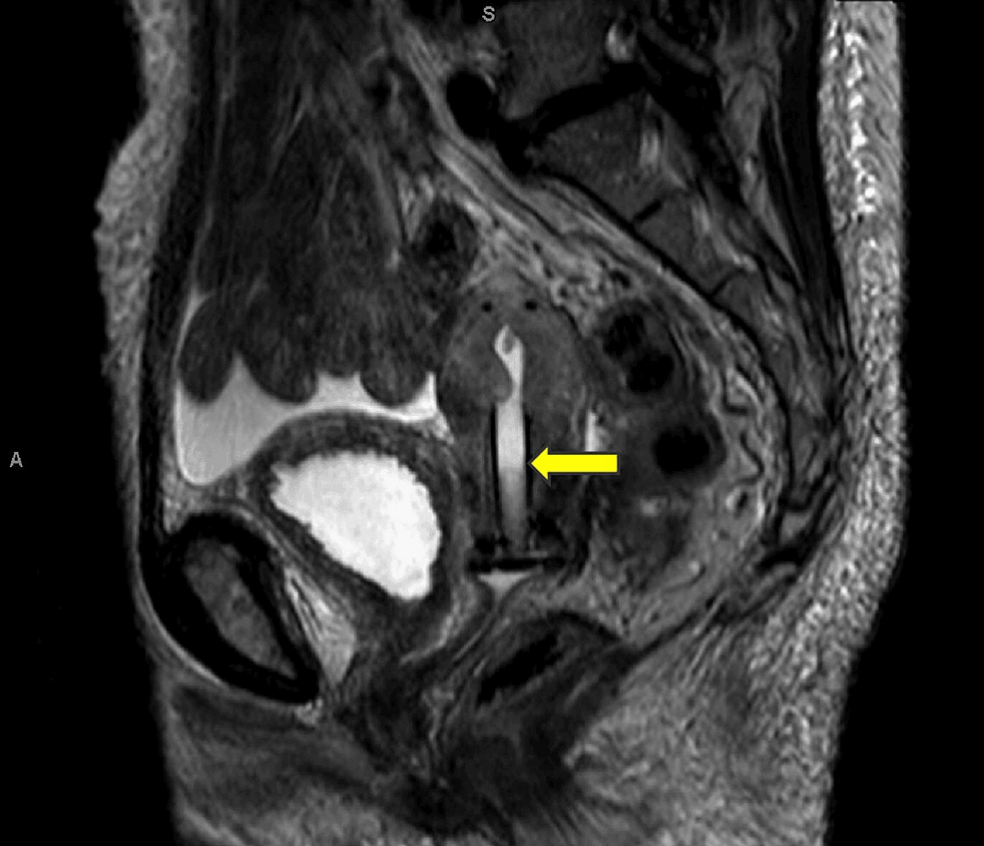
Sagittal MRI showing complete radiographic response after radiation and chemotherapy The cervical cancer is not visible but a Smit sleeve that was placed through the cervical os and into the uterus for brachytherapy is present (yellow arrow).
